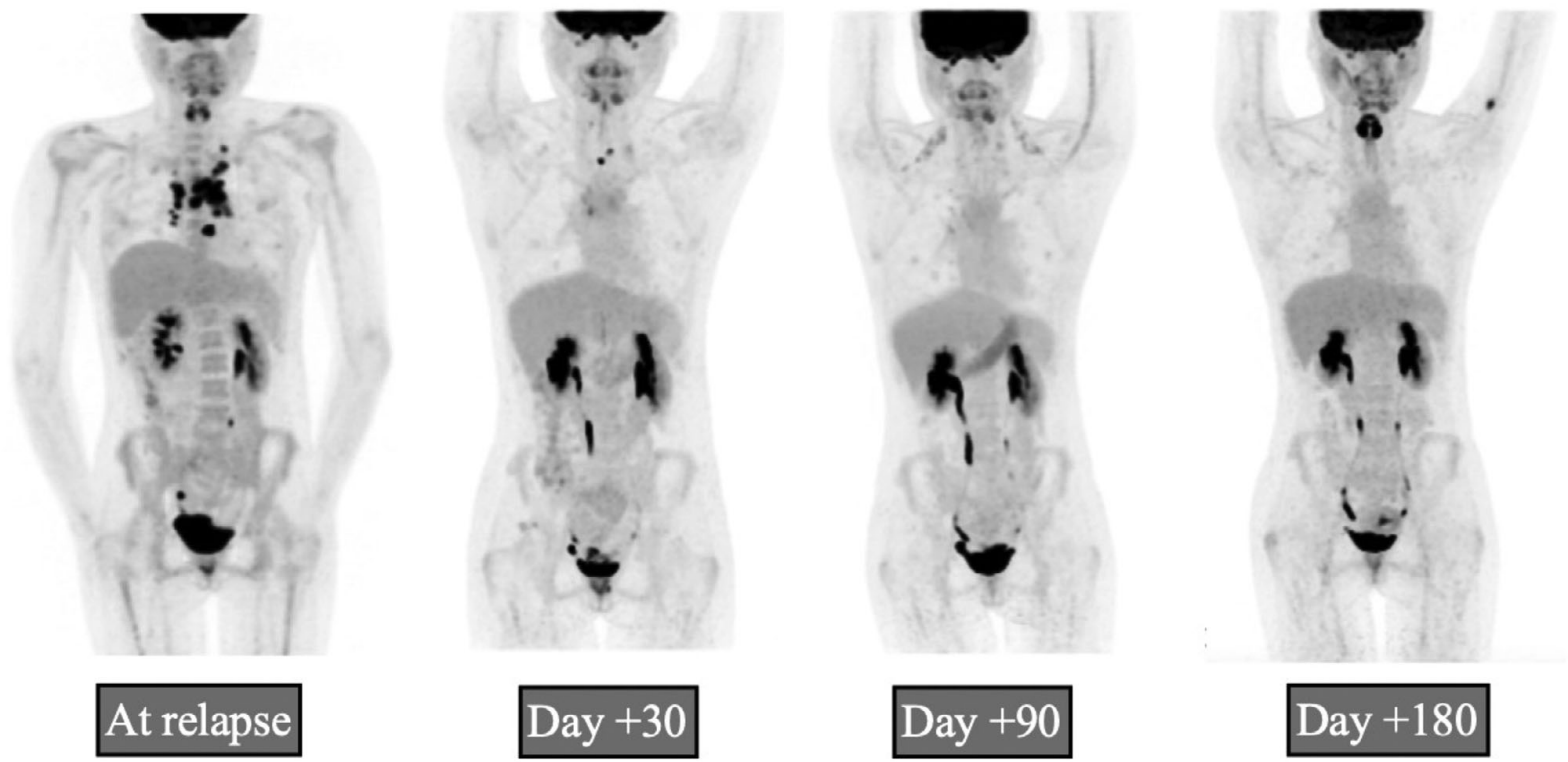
Clinical response at initial presentation and at days+30, +90, and +180 post‐treatment with lisocabtagene maraleucel. Positron emission tomography (PET) computed tomography (CT) scans at these timepoints demonstrate complete response per Lugano Classification lymphoma response criteria.
